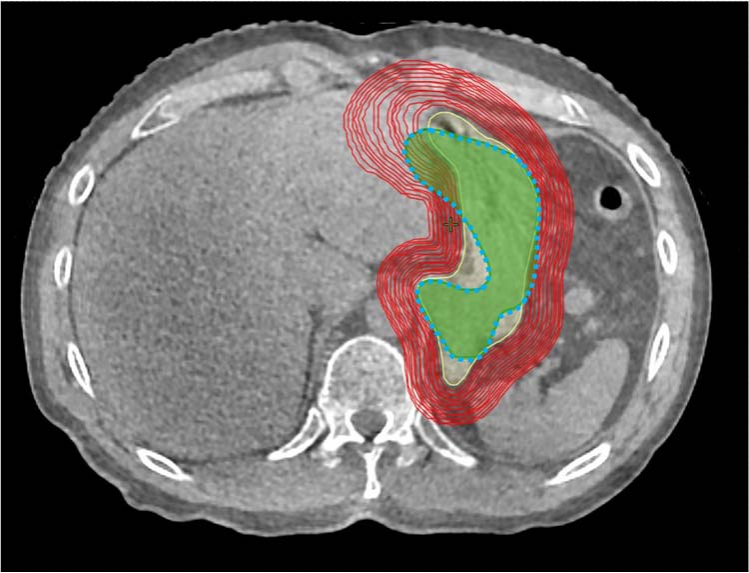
Transverse plane visualization of CTV on pre-treatment CBCT images, different planning target volume margins, and CTV contour on post-treatment CBCT (dashed line).
